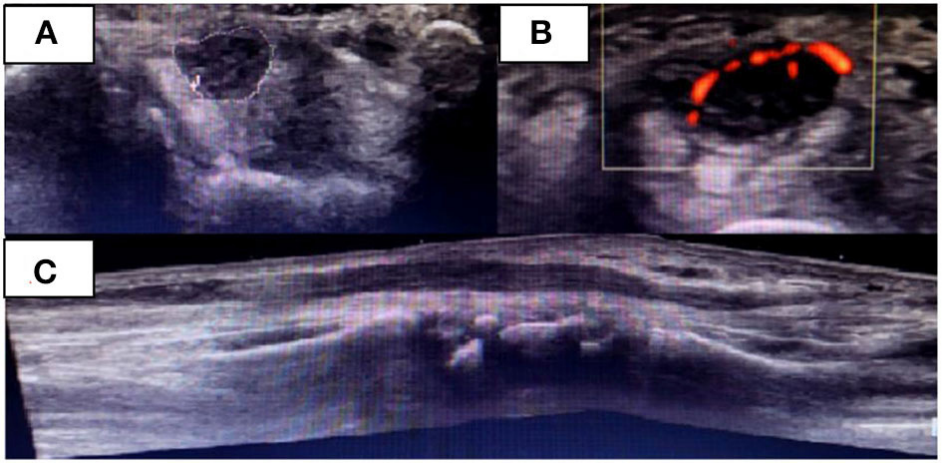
Right median nerve on the initial USG examination when median neuritis was diagnosed. Transverse (A, B) and (C) longitudinal nerve view in the carpal region. Presence of flow in the Power Doppler (B).
